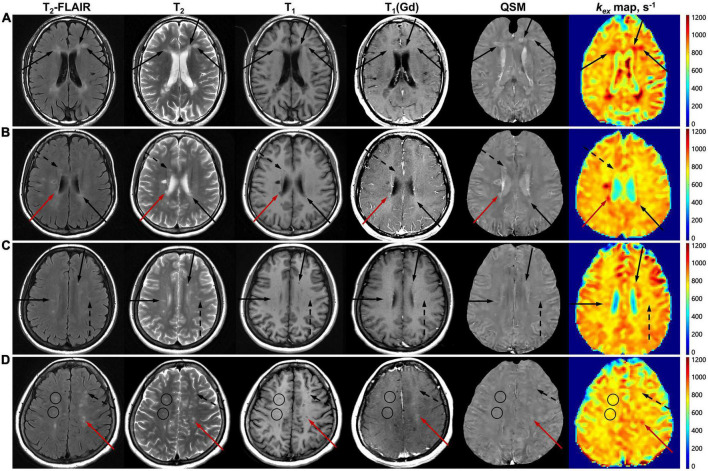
Gd^+^ lesions and the three patterns of Gd^–^ lesions on multimodal MRI. Images are from Patient No. 20 (A), No. 13 (B), No. 11 (C), and No. 27 (D), whose disease duration was 0.5, 1, 2, and 8 years, respectively. Gd^+^ lesions are indicated with red arrows (B,D). Pattern I of Gd^–^ lesions, which are *k*_*ex*_^+^ and QSM^+^, are indicated with black arrows (A–C). Pattern II of Gd^–^ lesions, which are either *k*_*ex*_^+^ and QSM^–^, or *k*_*ex*_^–^ and QSM^+^, are indicated with black dashed arrows (B–D). Pattern III of Gd^–^ lesions, which are *k*_*ex*_^–^ and QSM^–^, are indicated with black circles (D).
